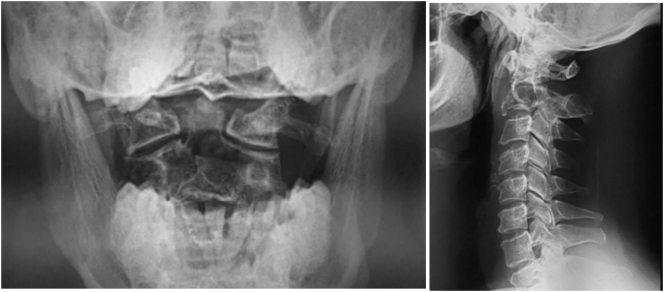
A lateral cervical spine radiograph with an open mouth view shows osteolysis of the odontoid process of the axis without signs of occipitocervical instability.
